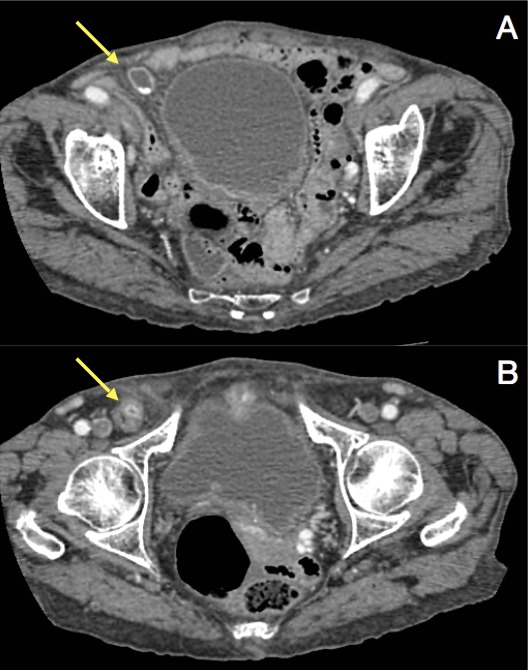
CT of the pelvis in the axial plane shows (A) the gallbladder body medially
